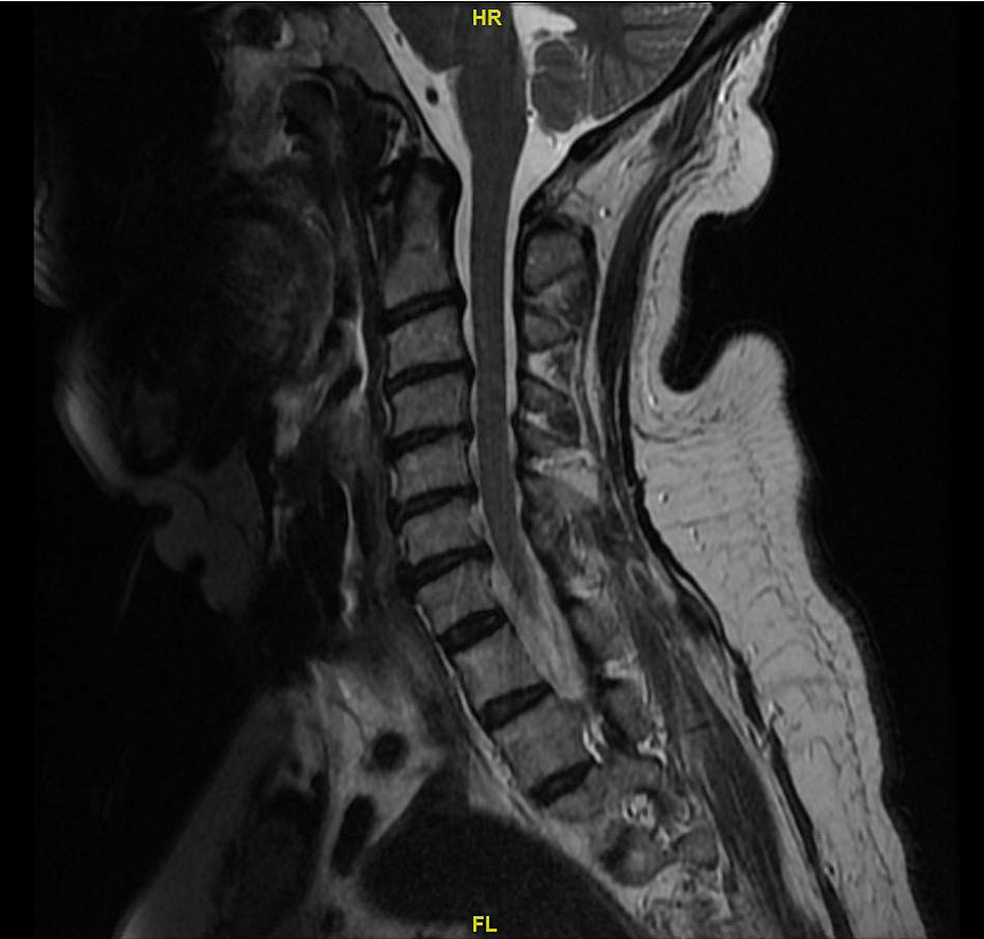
MRI cervical spine showing cervical radiculopathy
